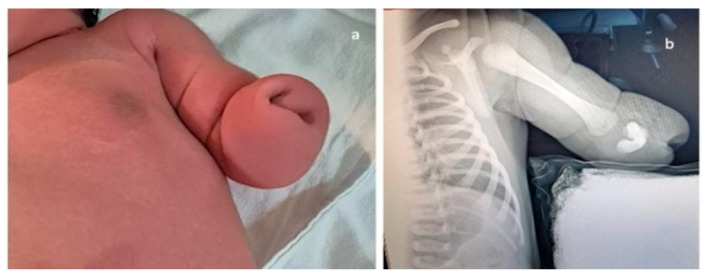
Upper-limb unilateral (left) reduction defect. In this case, of transverse limb deficiency, the defect was diagnosed at the end of the first trimester. Complete genetic work-up was performed, rendering normal results; the follow-up scans strengthened the suspicion of isolated anomaly, and the parents decided to continue pregnancy. (a)—postnatal aspect. (b)—radiography confirming the presence of the well-developed humerus and rudimentary buds of the radius and ulna. Multidisciplinary counseling was offered repeatedly, and led to surgical reconstruction and prosthetics, with good functional results.
